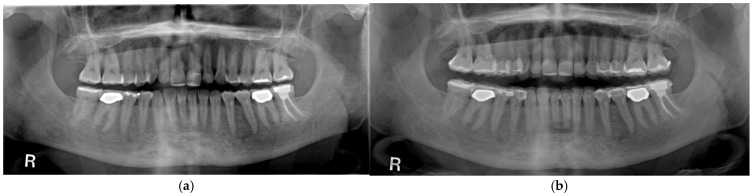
(a) Initial radiograph. (b) Final radiograph with CAD/CAM single crowns bonded onto unprepared teeth and pre-existing restorations.
